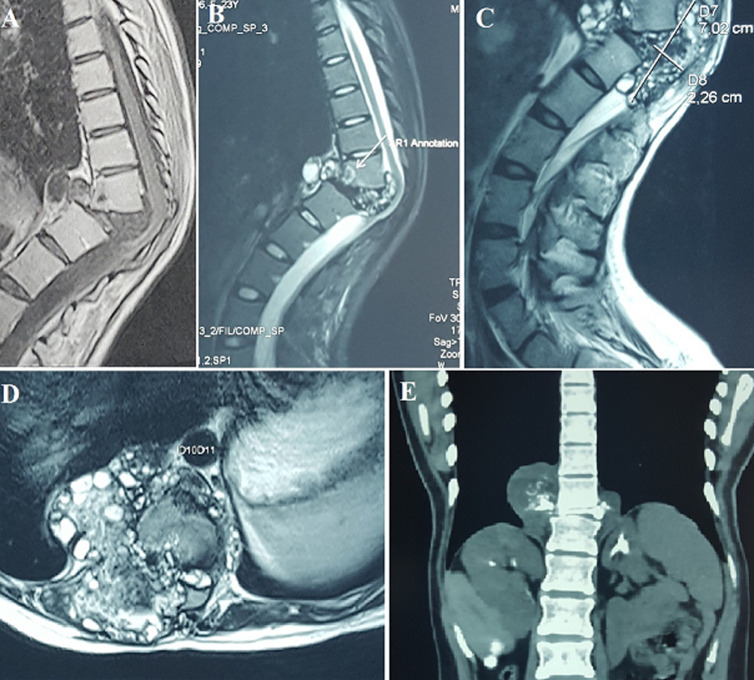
A) spinal MRI sagittal T1WI; B) sagittal T2WI; C) axial T2WI; D) frontal plane of CT spine; E) showing preoperative imaging
